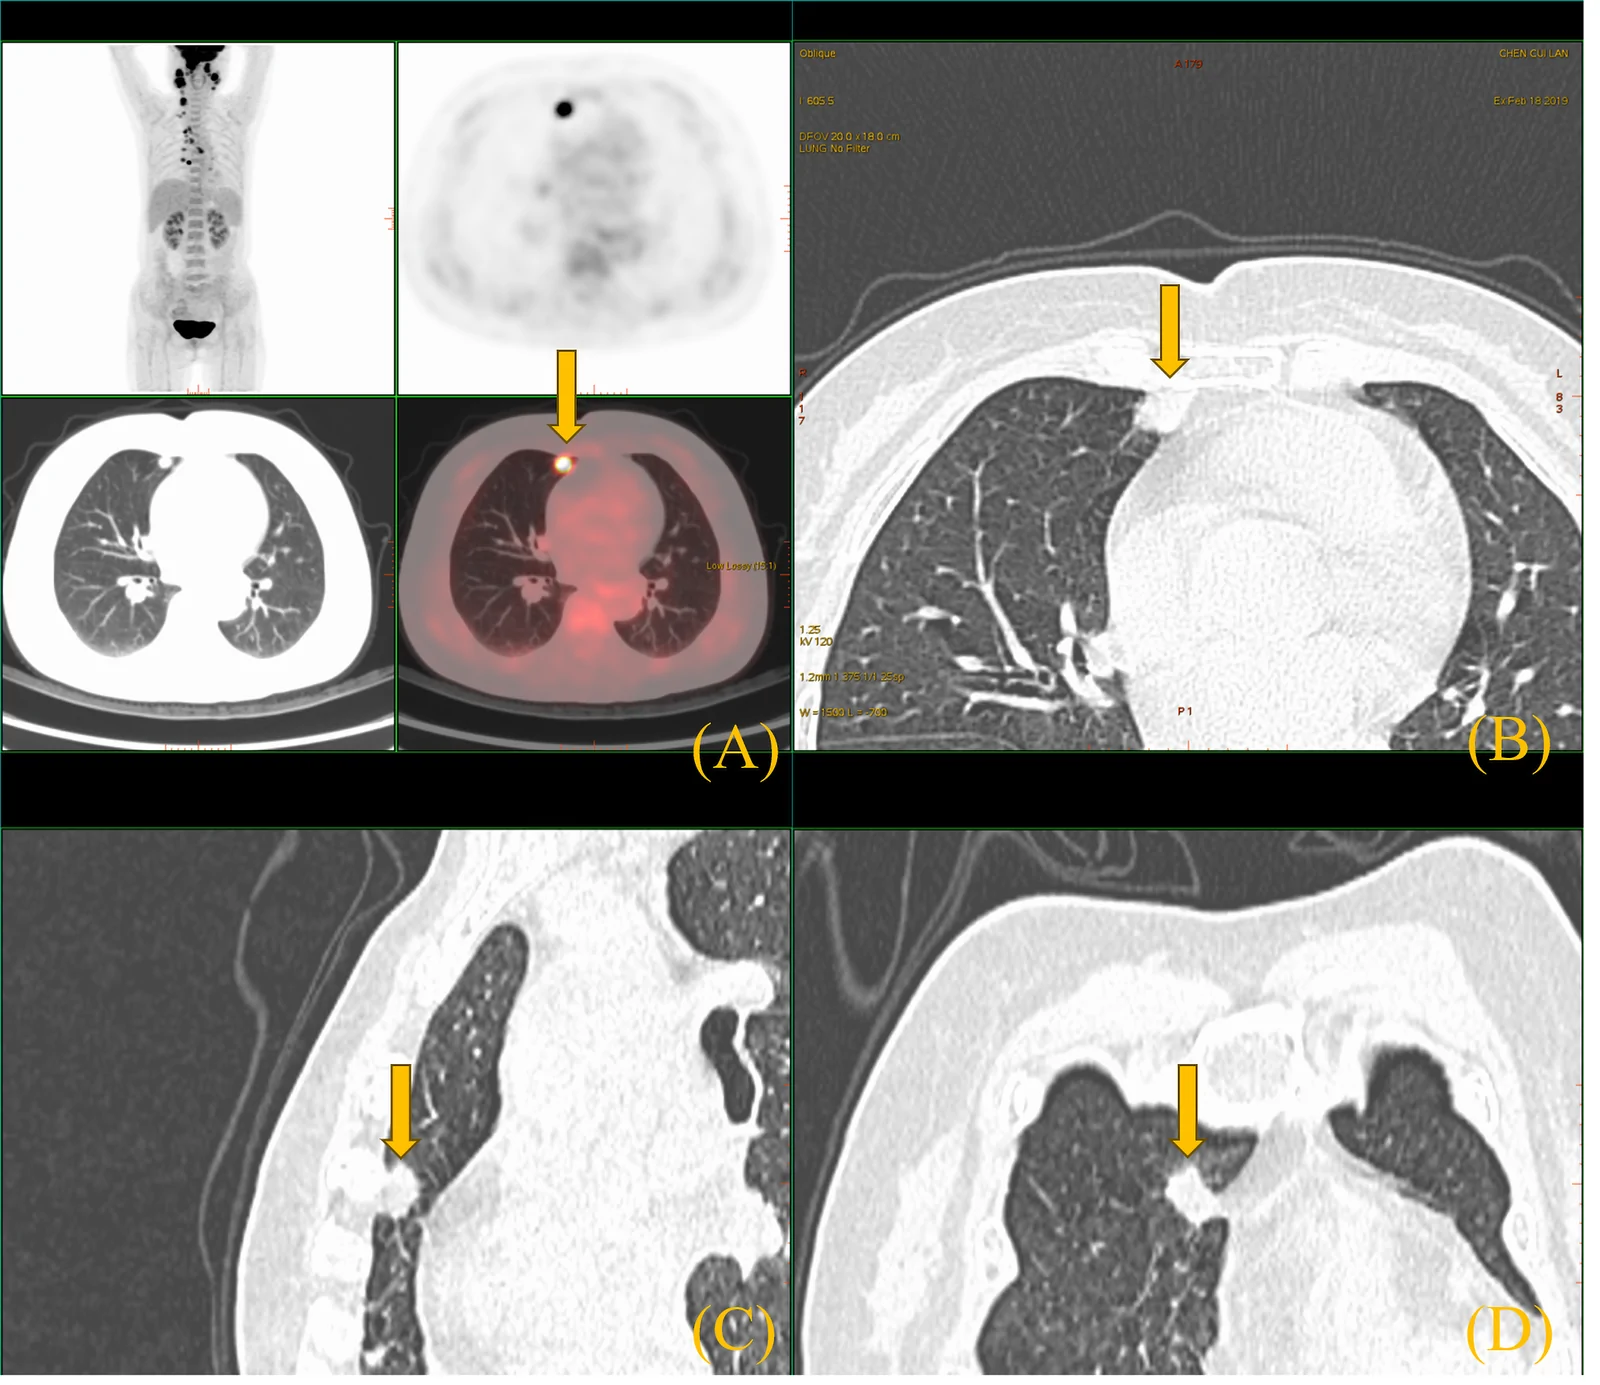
A 64-year-old female patient with undifferentiated carcinoma of the nasopharynx identified via nasopharyngeal endoscopy underwent ¹^8^F-FDG PET-CT (A), which revealed a solitary irregular nodule in the anterior segment of the right upper lung lobe (indicated by the yellow arrow) with increased FDG uptake (SUVmax = 12.38). The lesion showed ill-defined borders with the adjacent mediastinal pleura. Thin-section 1.25 mm CT images in axial (B), sagittal (C), and coronal (D) planes demonstrated the irregular morphology of the nodule, which appeared less rounded than typical metastatic lesions. An experienced physician with over 20 years of expertise in ¹^8^F-FDG PET-CT interpretation considered the evidence insufficient to definitively diagnose the nodule as a metastasis at the time of examination. The MLP model (integrating Deep + Radiomics(T) + clinical features + SUVmax) calculated the probability of distant metastasis in this patient as 0.9999999997. The MLP-based hybrid model may serve as auxiliary reference for preliminary risk screening of occult SDM.
